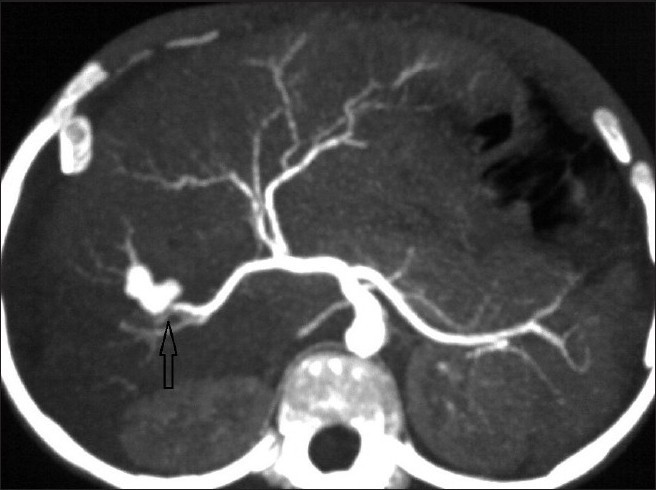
Axial plane thick multiplanar reformation CT angiography image showing pseudoaneurysm of the right branch of the hepatic artery (upward arrow)
